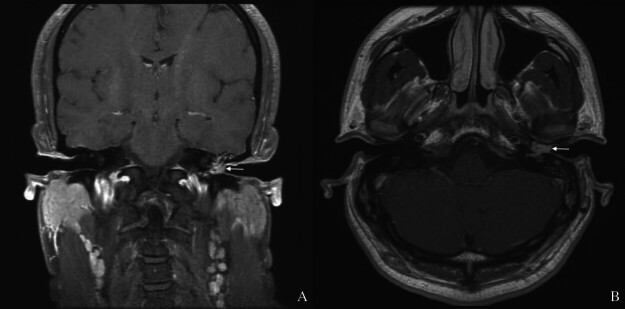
Magnetic resonance imaging showing left middle ear and canal enhancement. (
